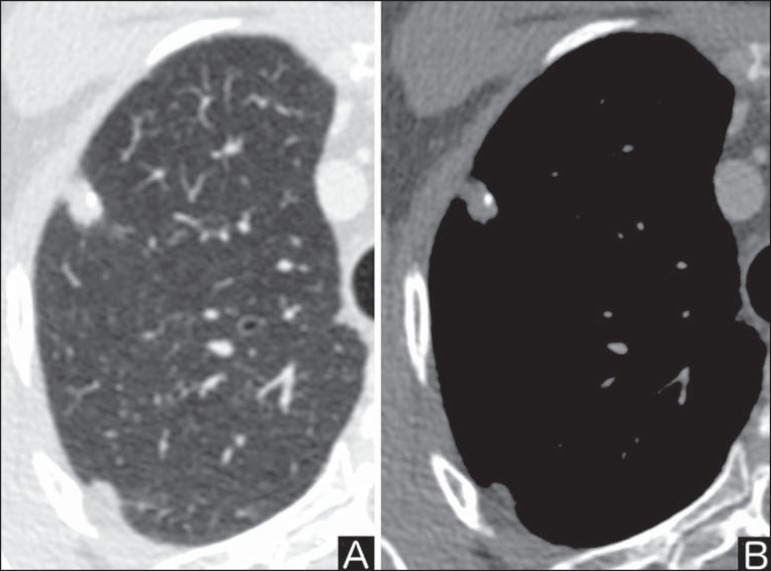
Malignant calcification pattern.* Chest CT - lung
